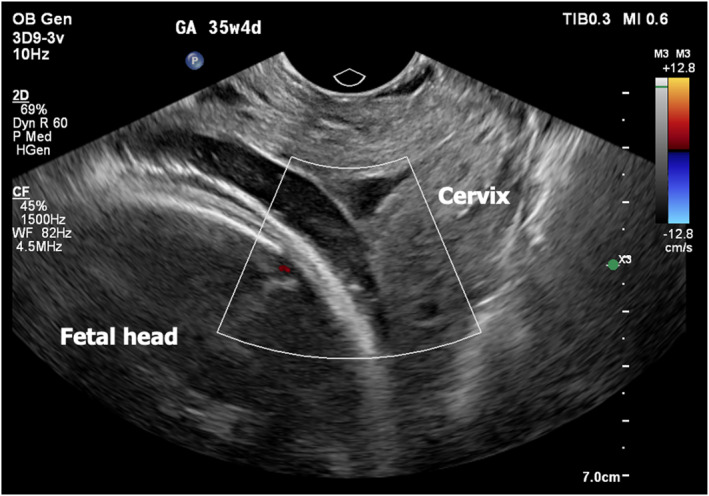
Postsurgical transvaginal ultrasonography showing complete resolution of the type II vasa previa.
